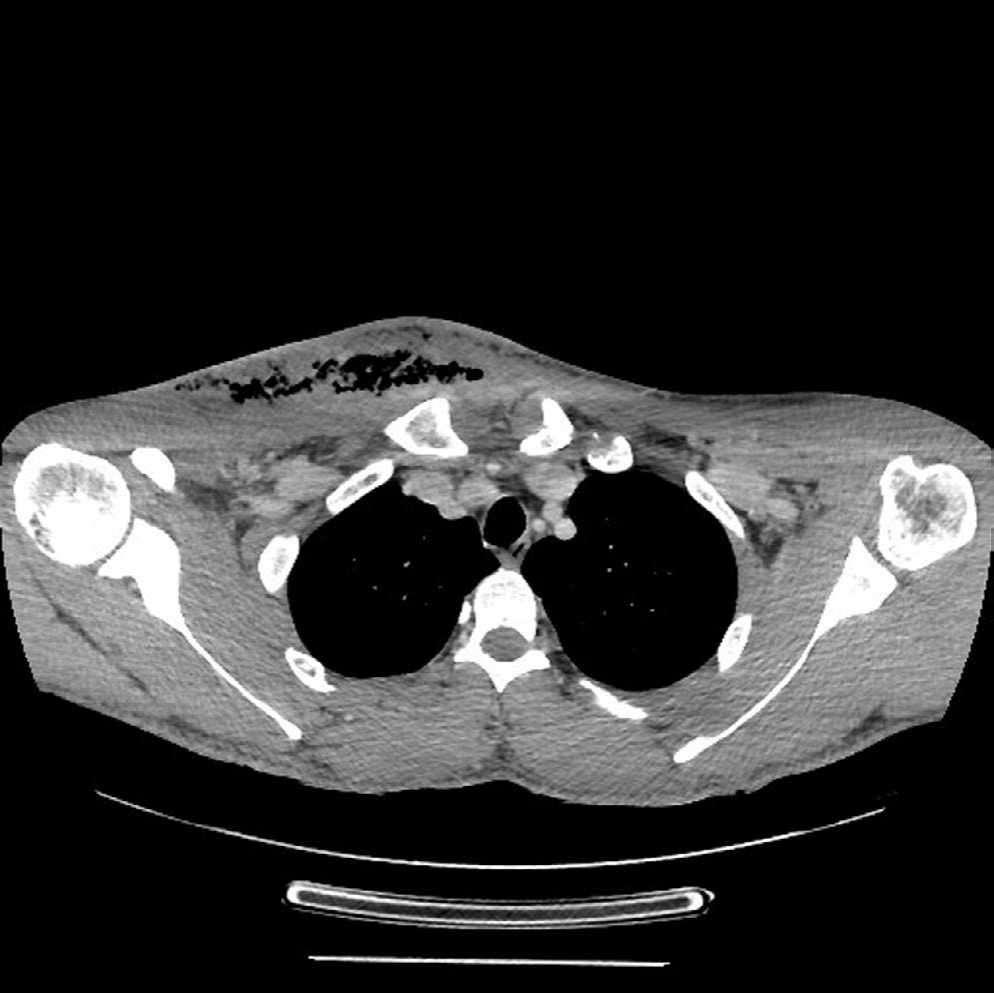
Pre‐operative CT scan of the patient's upper thorax
